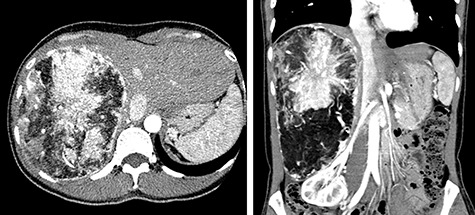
Abdominal CT on admission; the tumor had enlarged, occupying the entire right lobe of the liver, and measuring 200 × 140 × 120 mm.
